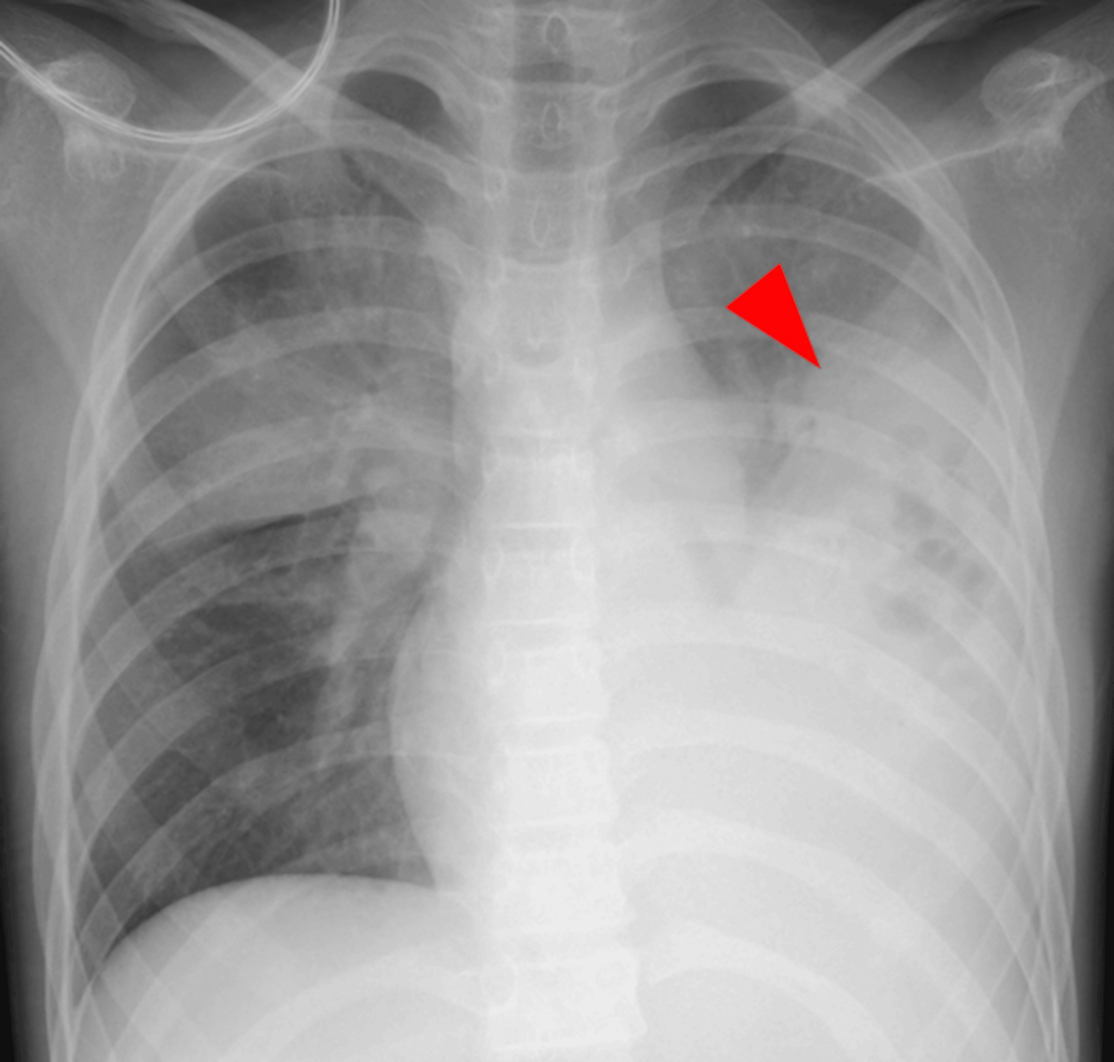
Chest X-ray taken four days before transfer to our pediatric intensive care unit. Chest X-ray revealed lobar pneumonia (arrowhead).
